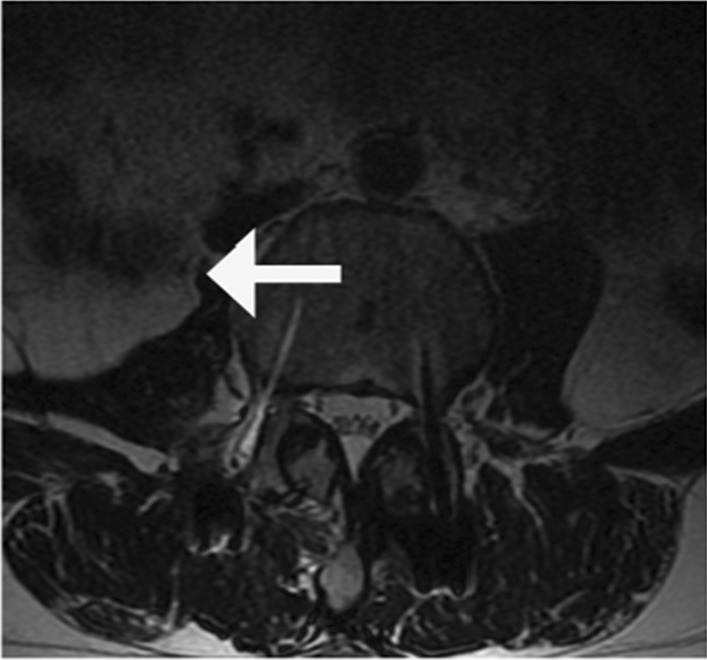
The abdominal MRI showed that the abdominal hematoma was not completed absorbed
